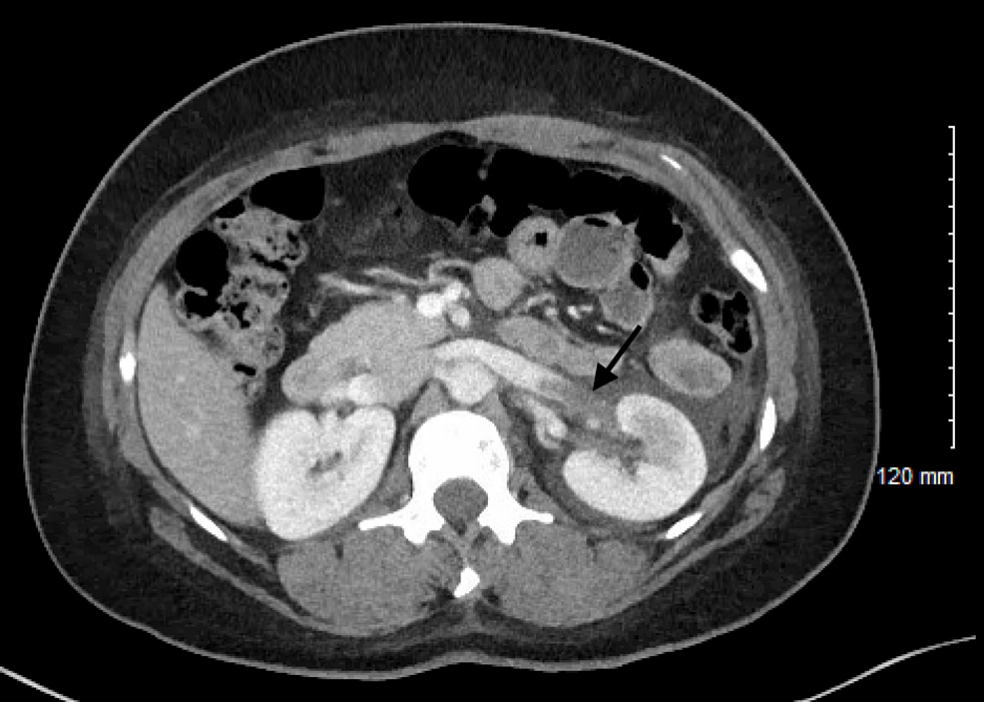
Computed tomography (CT) abdomen and pelvis with contrast showing thrombosis of the left renal vein (arrow)
